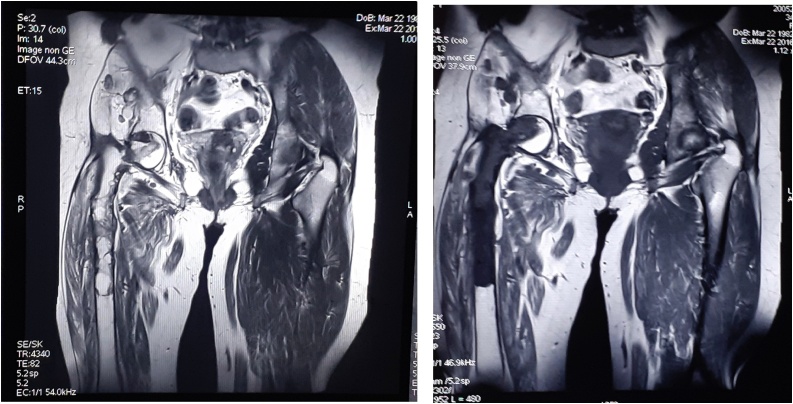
MRI images to evaluate the local extension of cystic lesions.
